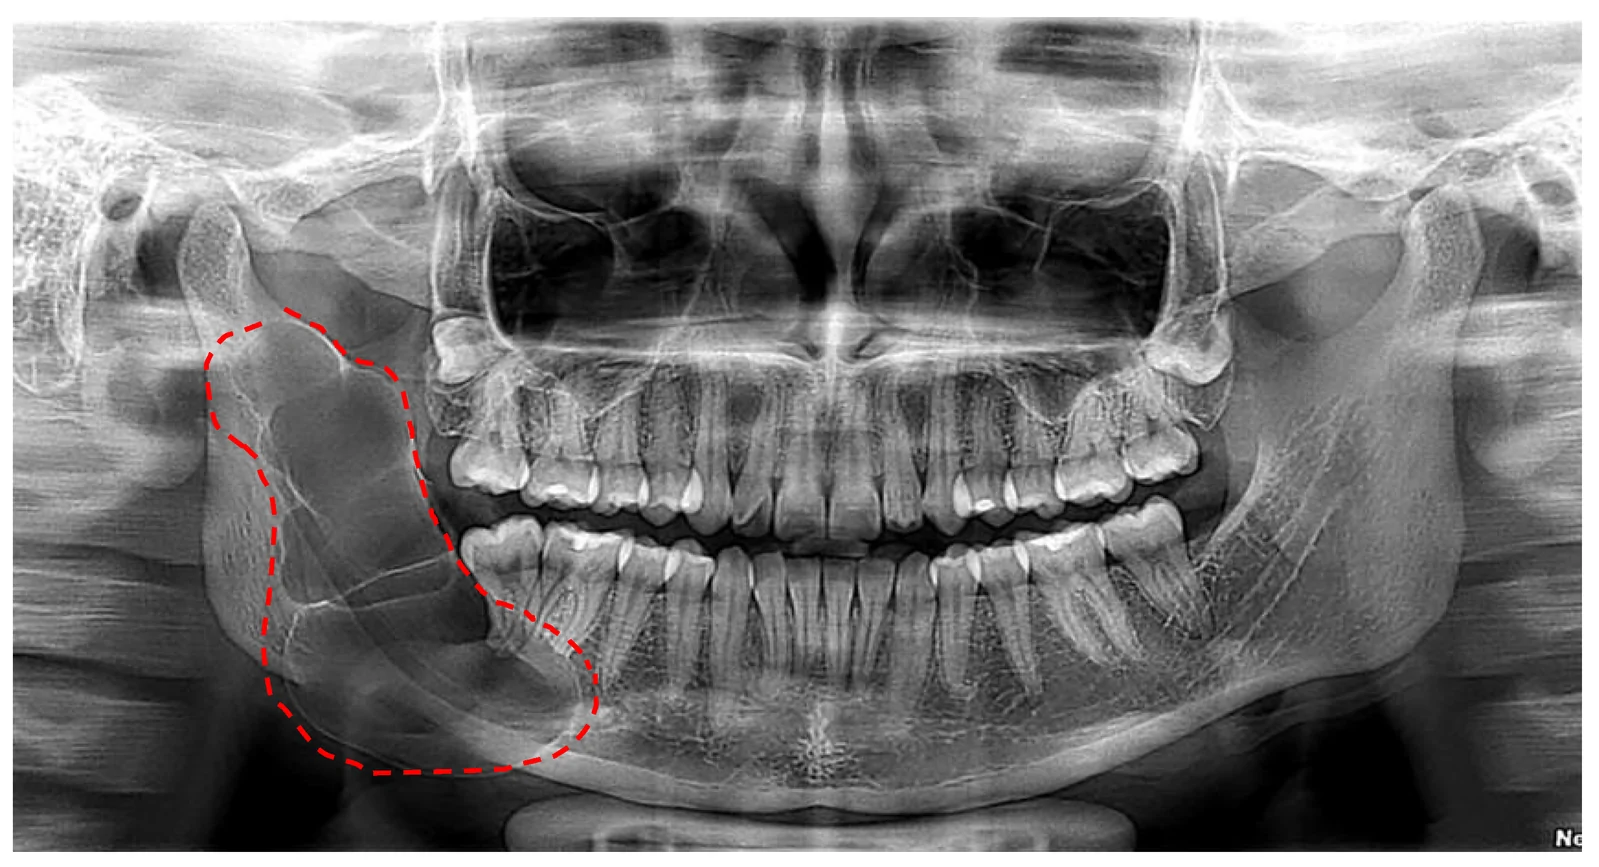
Initial panoramic radiograph. The dashed red outline delineates a unilocular radiolucent lesion with well-defined corticated margins extending from the mesial surface of the right mandibular second molar through the mandibular ramus to the ipsilateral coronoid process.
